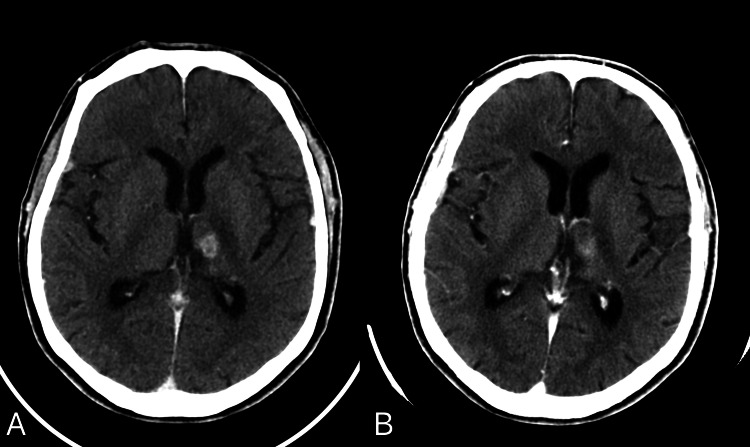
Follow-up contrast-enhanced CT scans of the brain done almost 6 months (A) and 1 year (B) post initiation of anti-TB treatment showing the significant reduction in size of the left thalamic lesion, and near resolution of the surrounding edema and mass effect. Only a faint patchy enhancement remained on the 1-year post-treatment follow-up image (B). CT: computed tomography; TB: tuberculosis
